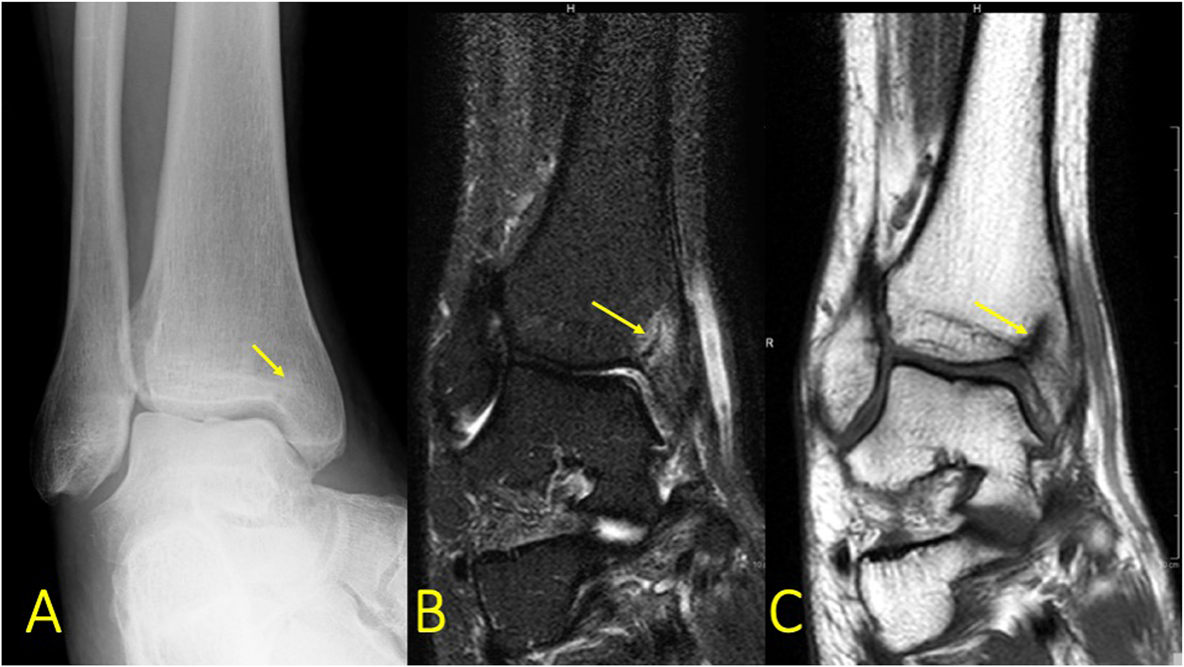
A 57-year-old male with pain in the medial malleolus without a history of trauma. a Radiograph, mortise view, b PD FS coronal image, and c T1-weighted coronal image. MRI showed an occult fracture of the medial malleolus (arrow) which in retrospect was visible on the radiographs. There is a minor irregularity of the cartilage signal in the subchondral central part of the talar trochlea, probably after previous injuries
